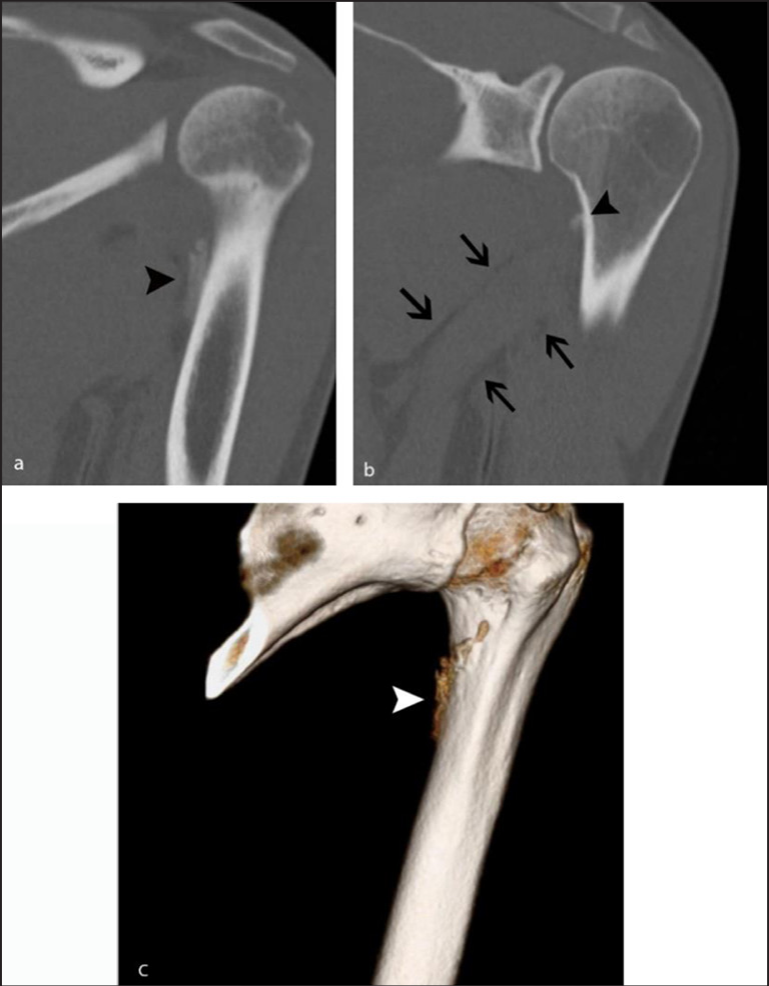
Follow-up CT five weeks later. a) Oblique coronal reformatted image of the proximal humerus shows a slightly more dense aspect of the periphery of the calcification (black arrowhead). b) MIP reconstructions (1.5 mm) clearly show that the calcification is located at the medial crest of the lesser tuberosity (black arrowhead). Note also the perimuscular fat pads surrounding the teres major muscle (black arrows). c) Volume Rendering Technique (VRT) image revealing irregular contour (white arrowhead) near the medial crest of the lesser tuberosity.
